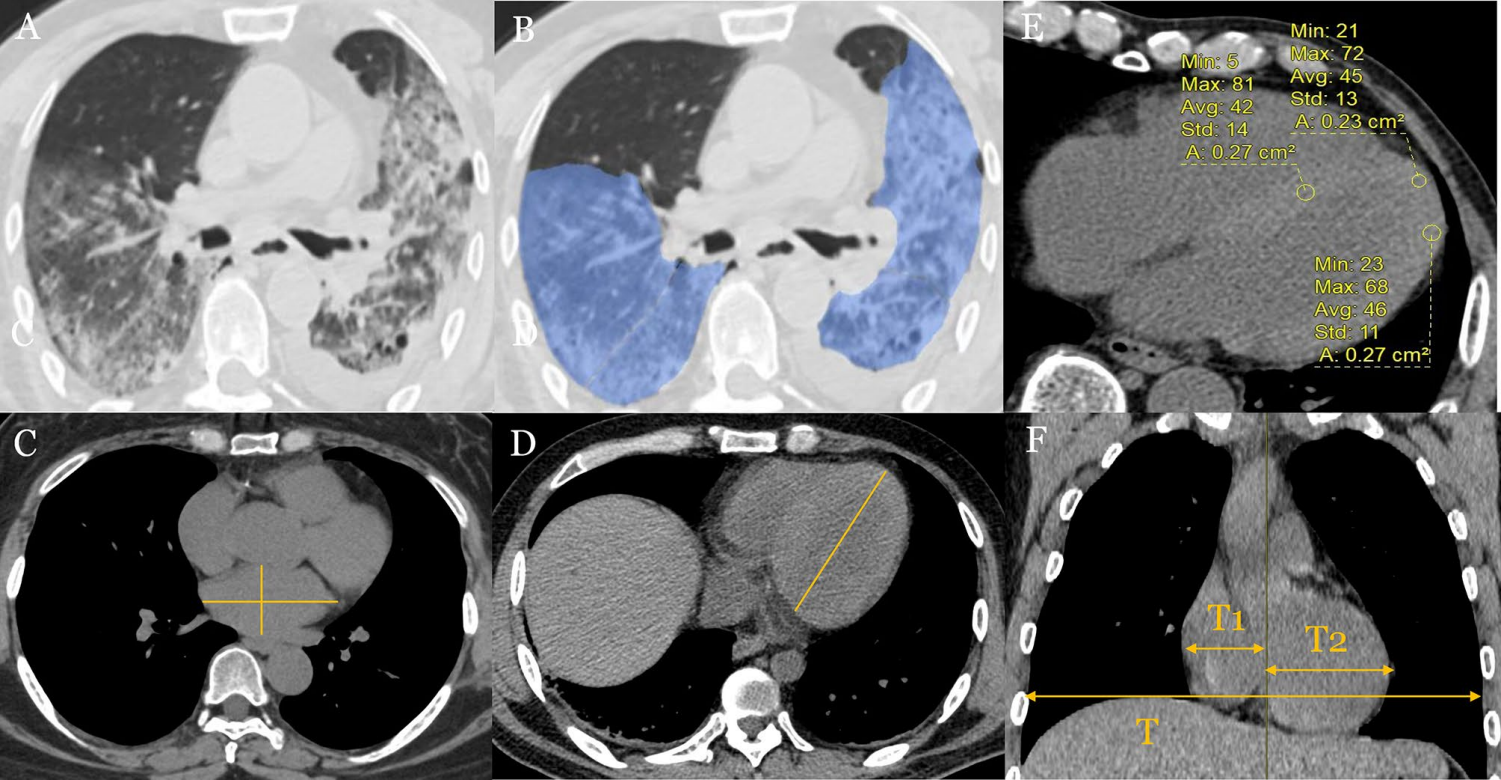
(A) A 74-year-old man with dyspnea, severe pneumonia. An axial CT image showed diffuse large regions of GGO, partial consolidation and pleural effusion. (B) Post-processing software showed the area and percent of GGO and consolidation. (C) Measurement of the anteroposterior diameter of LA and left and right diameters of LA. (D) Measurement of long diameter of LV. (E) Measurement of myocardial CT value. (F) Measurement of CTR (T1 + T2/T).
